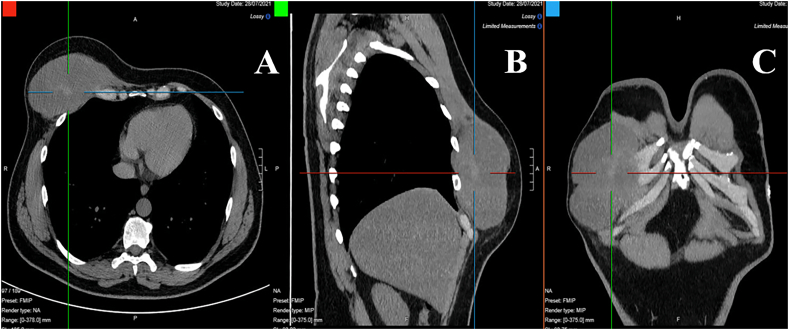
A,B,C. Thoracic contrast-enhanced computed tomography (CECT) showing a smooth contour, heterogeneous and hypodense subcutaneous soft tissue mass, anterior to the right pectoral muscles and to the right 4th–7th rib (panel A axial view, panel B sagittal view, panel C coronal view).
